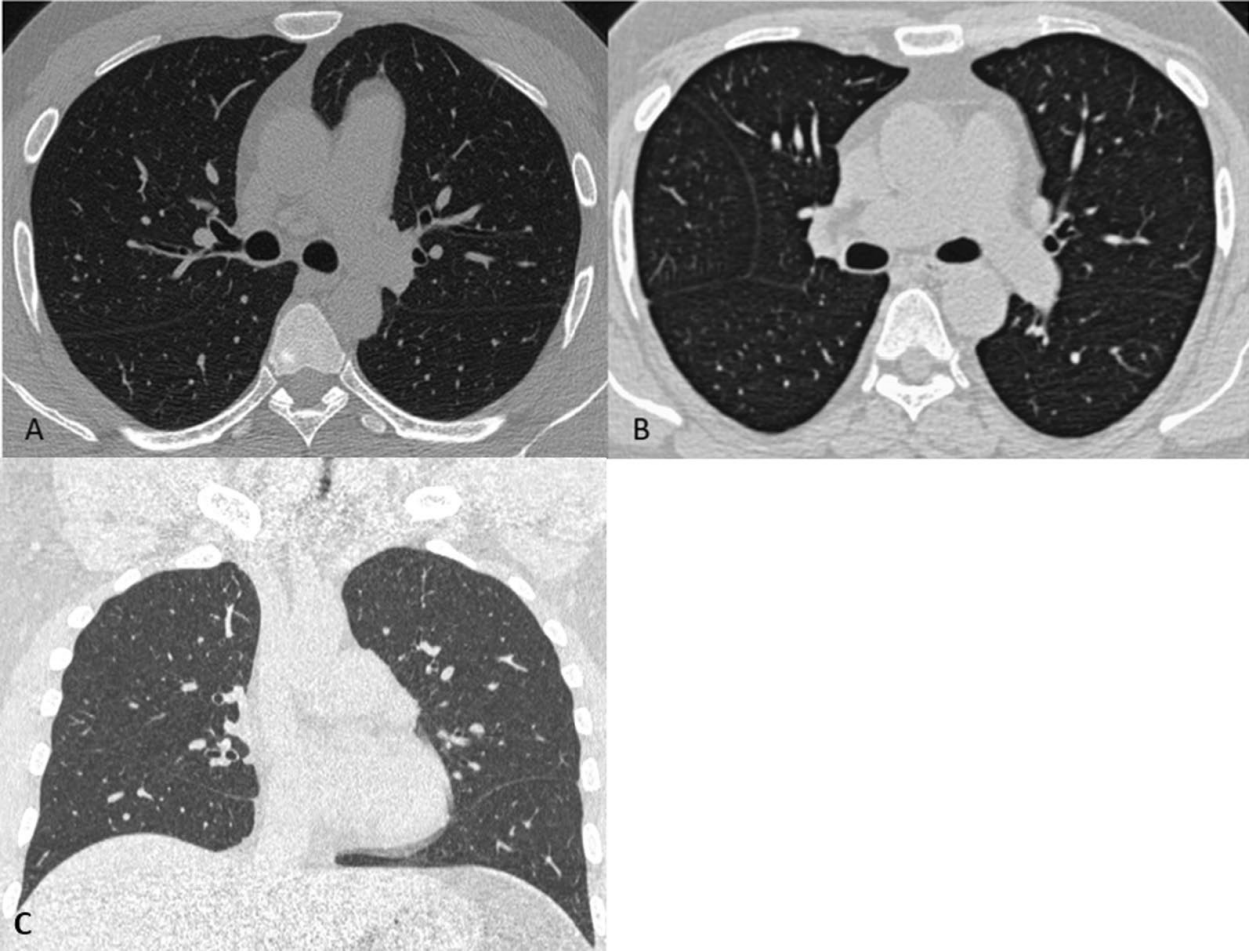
CT axial images seen are comparisons of pericardial agenesis (right) with normal CT chest (left). A In the absence of the pericardium, lung tissue can be detected between the aorta and the main segment of the pulmonary artery. B Normally, the aortopulmonary window is covered by pericardium and contains some fat. C Lung window—CT coronal image reveals an area of lung parenchyma between left hemidiaphragm and the base of the heart
